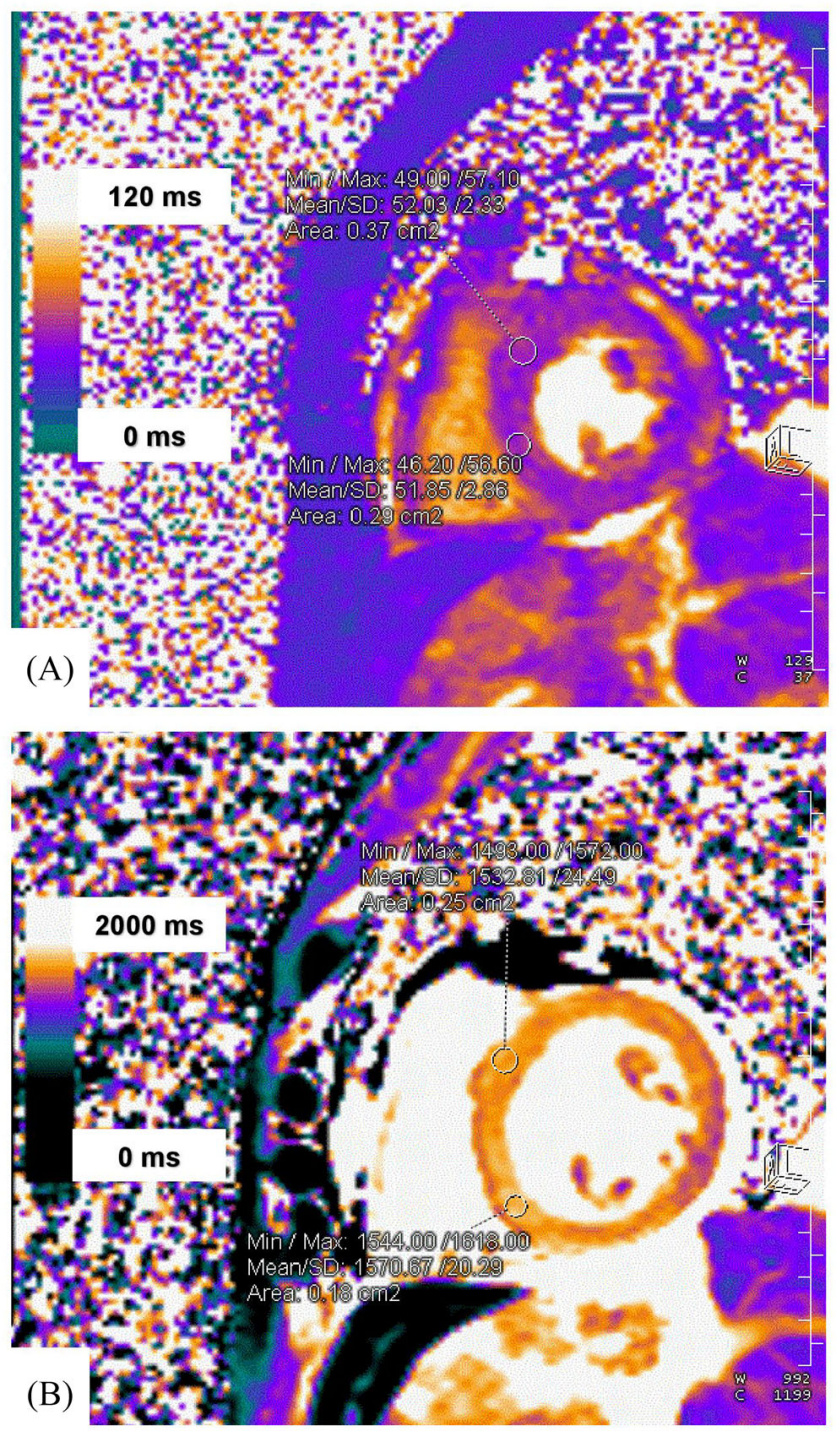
Cardiac MRI native T1 and T2 maps with color scale (obtained on a Siemens Vida 3‐Tesla MRI scanner) of patient 2 is shown above. Native T2 values (A) were increased throughout the myocardium with several values greater than 50 ms (upper limit normal 38 ± 3.5 ms). Native T1 values (B) were also diffusely increased with several values greater than 1550 ms (upper limit normal 1227 ms). The increased T1 and T2 values were most prominent in the segments with regional wall motion abnormalities (basal and mid segments of the septum and anterior walls), and no delayed enhancement was appreciated following IV contrast. These findings were most consistent with the diagnosis of stress cardiomyopathy
